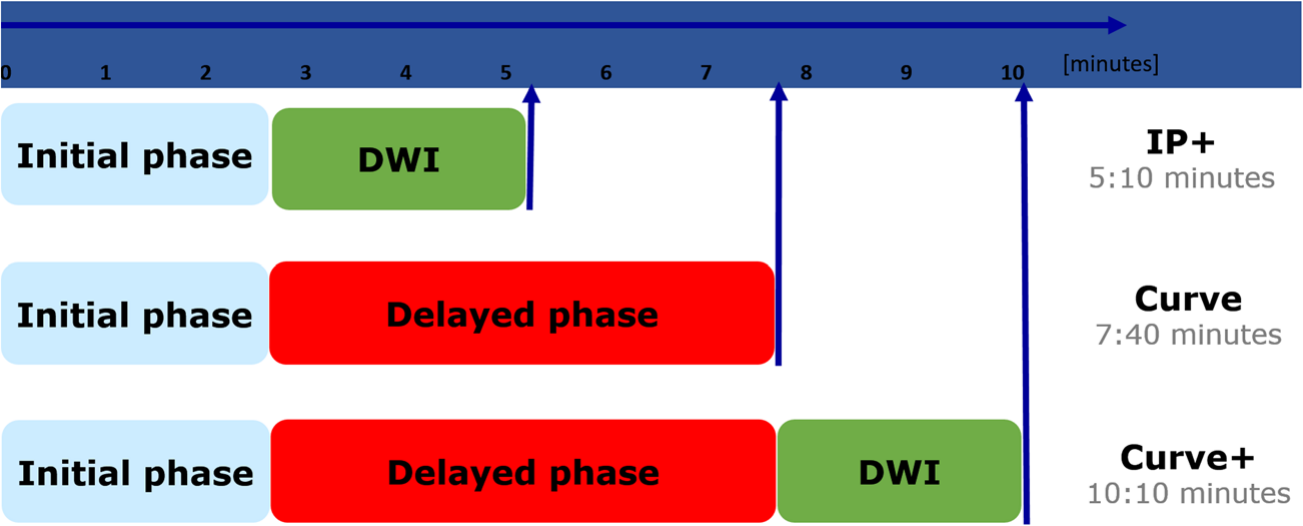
Graphical summary of the three protocols investigated in the present study. The combination of the initial phase (IP) and DWI gave IP+. Requiring a scanning time of 5:10 min, IP+ enables assessment of the initial phase, lesion morphology, and the ADC. The combination of the initial phase (IP) and the delayed phase gave Curve. Requiring a scanning time of 7:40 min, Curve enables assessment of the initial and the delayed phase (washout, plateau, and persistent increase). Integration of DWI into Curve gave Curve+ (scanning time 10:10 min). A potential alternative to be investigated in the future would be interleaved curve: It adds one scan of the DP to the IP+ (scanning time 6:10 min) and hereby combines the potential of Curve+ within a much shorter examination time
